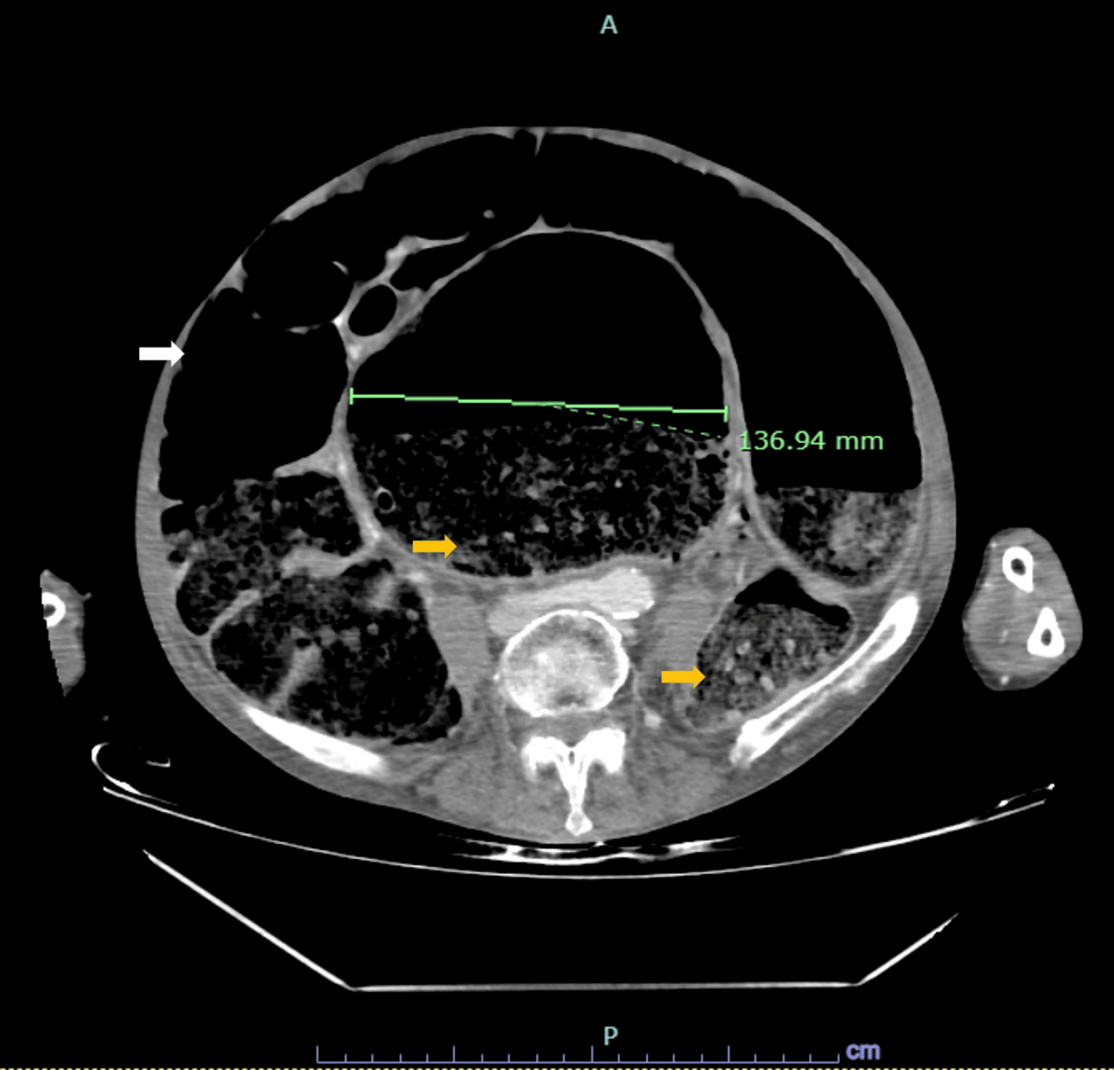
Axial CT findings in acute colonic pseudo-obstruction. Axial computed tomography (CT) image demonstrating marked colonic dilation (white arrow), with a measured transverse diameter of approximately 13.7 cm, and extensive fecal impaction consistent with a large rectal fecaloma (yellow arrows), in keeping with acute colonic pseudo-obstruction (Ogilvie syndrome).
